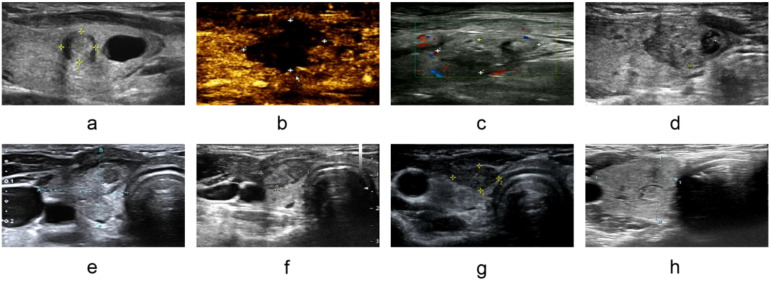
Ultrasonograms of a 24-year-old female patient with 24 months of follow-up. (a) Preoperative, (b) Contrast-enhanced ultrasound immediately after ablation, (c) 1 day postoperatively, (d) 1 month postoperatively, (e) 3 months postoperatively, (f) 6 months postoperatively, (g) 12 months Postoperatively, (h) 24 months Postoperatively.
